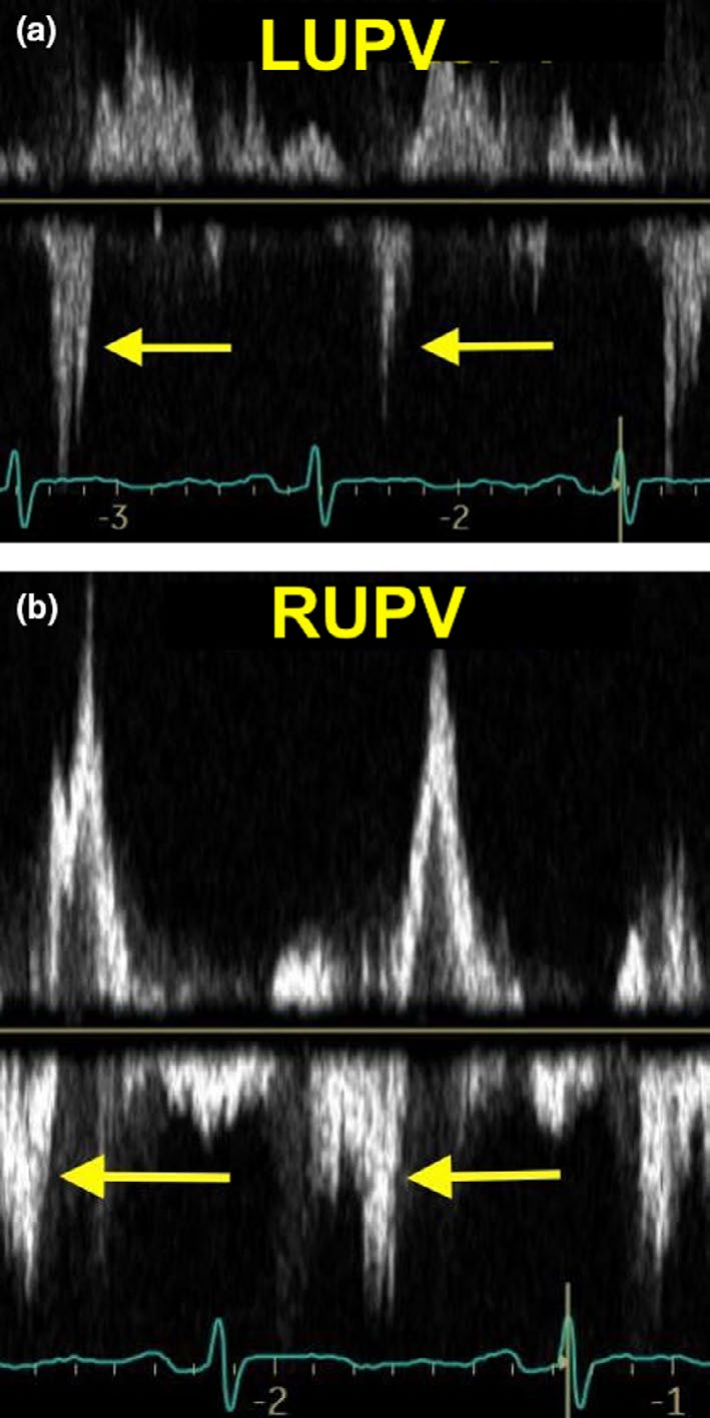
Pulsed‐wave Doppler recording revealing bilateral pulmonary venous systolic flow reversal A, Left upper pulmonary vein (LUPV). B, Right upper pulmonary vein (RUPV)
